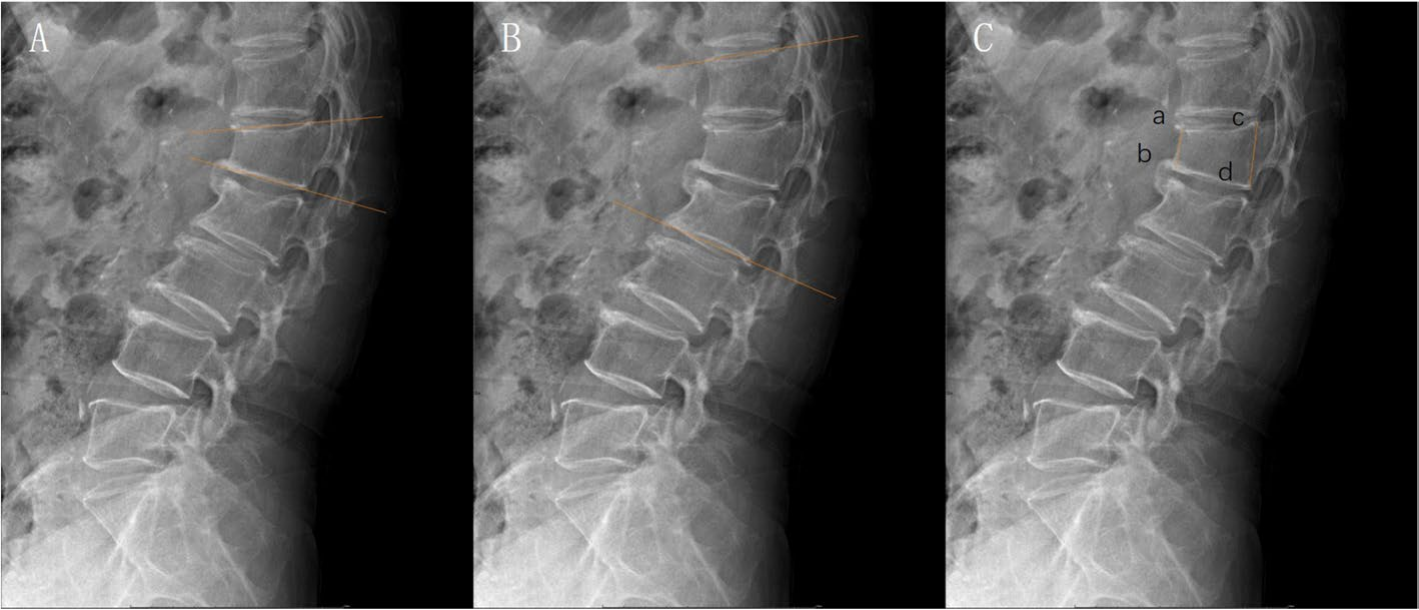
Preoperative X-ray imaging. A kyphosis angle; B Cobb’s angle; C vertebral compression degree (cd-ab/cd)
